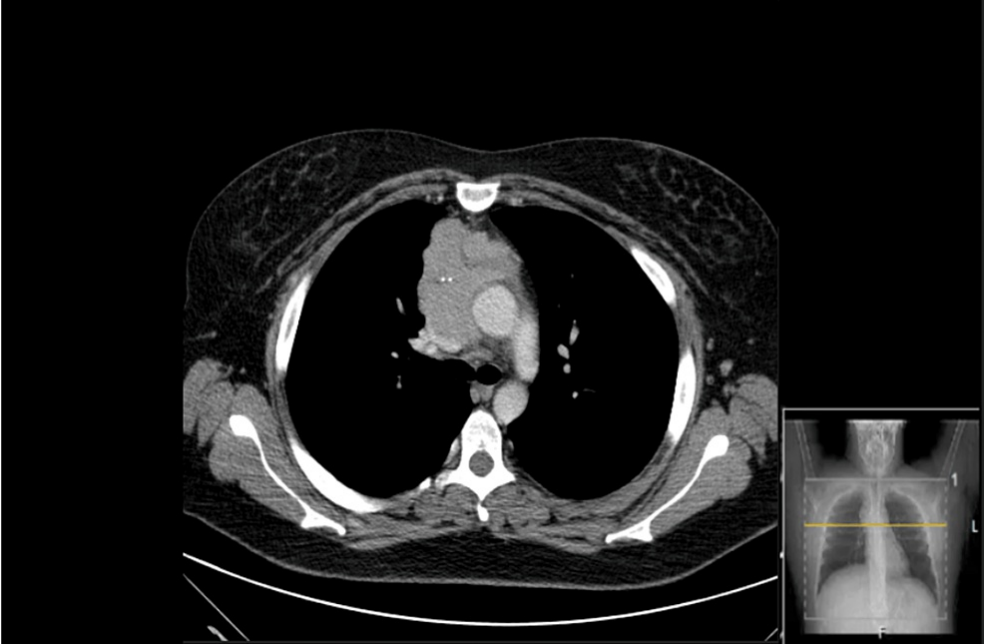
CT-Chest showing a heterogeneous anterior mediastinal solid lesion (7.9x6.7x6.2cm), with areas of central hypocaptation and millimetre calcification, along with lobulated contours, resulting in a significant reduction in the caliber of the distal strand of the left brachiocephalic vein, superior vena cava and contact with the right atrial appendage.
